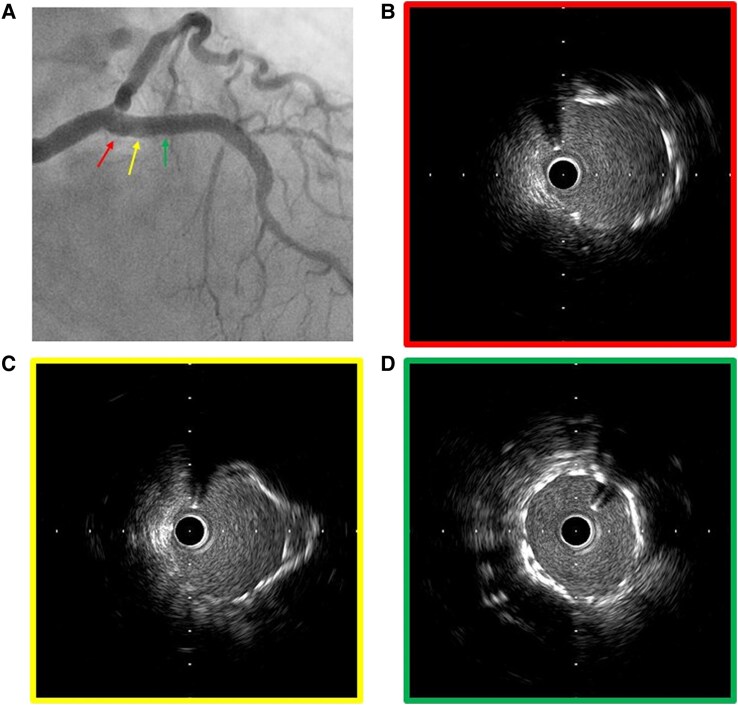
(*A*) Final coronary angiography following stent implantation. (*B–D*) Intravascular ultrasound images obtained sequentially from the proximal to distal segments within the proximal left anterior descending, confirming drug-eluting stent deployment in the true lumen at the site where the wire had migrated into the subintimal space. Post-dilatation resulted in good symmetric stent expansion and acceptable lumen gain.
